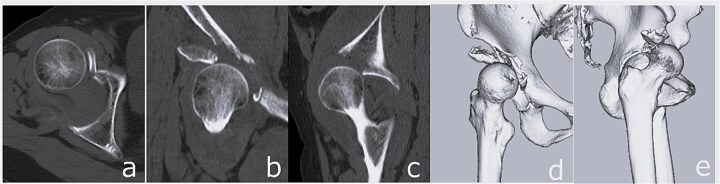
CT images obtained at initial presentation: Axial (a), coronal (b), and sagittal (c) views, as well as three-dimensional reconstructions (d and e), showing anterior hip dislocation with fractures of the acetabular anterior wall and anterior inferior iliac spine.
